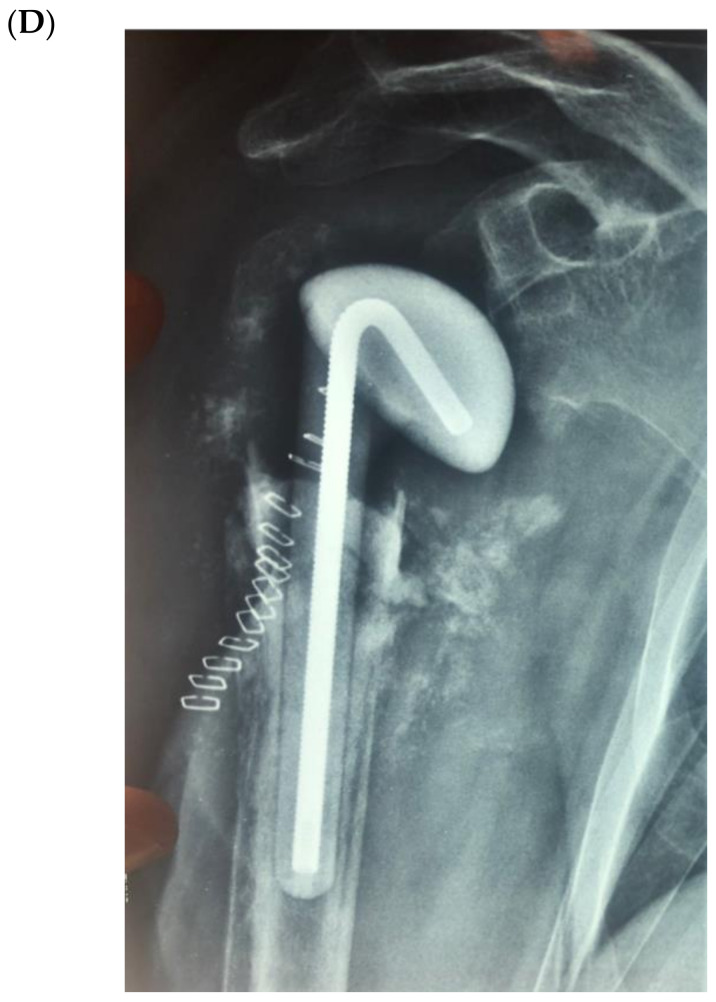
(A) The X-ray of a case of an infected proximal humerus fracture sequelae, originally treated with a plate. (B) After plate removal, the first RSA was implanted, which subsequently dislocated, resulting in infection. (C) A two-stage procedure was performed: removal of the RSA, debridement and implant of a cement antibiotic spacer with vancomycin. (D) Spacer X-ray postoperative image.
